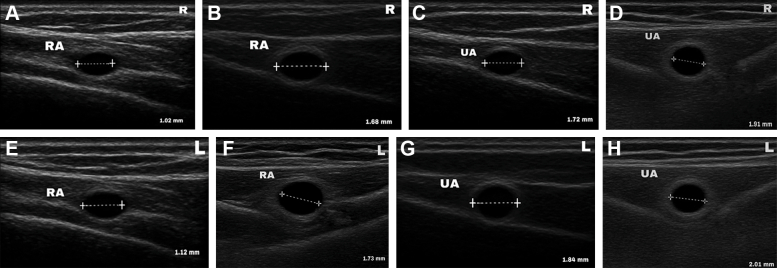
Arterial Ultrasonography Showing the Difference in Radial and Ulnar Artery Size Between Day 1 (Pretreatment) and Day 3 (Follow-Up) Normal sizes are 2.325 ± 0.4 mm (radial artery) and 2.358 ± 0.39 mm (ulnar artery).^7^ (A and B) Comparison of right distal radial artery between (A) pre- and (B) post-treatment showed the artery diameter increase 64.7% after therapy. (C and D) Comparison of right distal ulnar artery between (C) pre- and (D) post-treatment showed the artery diameter increase 11.1% after therapy. (E and F) comparison of left distal radial artery between (E) pre- and (F) post-treatment showed the artery diameter increase 54.5% after therapy. (G and H) comparison of left distal ulnar artery between (G) pre- and (H) post-treatment showed the artery diameter increase 9.24% after therapy. RA = radial artery; UA = ulnar artery.
